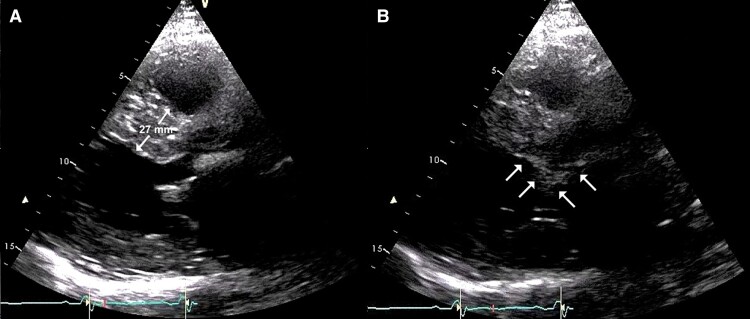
Echocardiogram shows (*A*) significant thickness of the interventicular septum and (*B*) systolic anterior motion of the anterior mitral leaflet (arrows).
